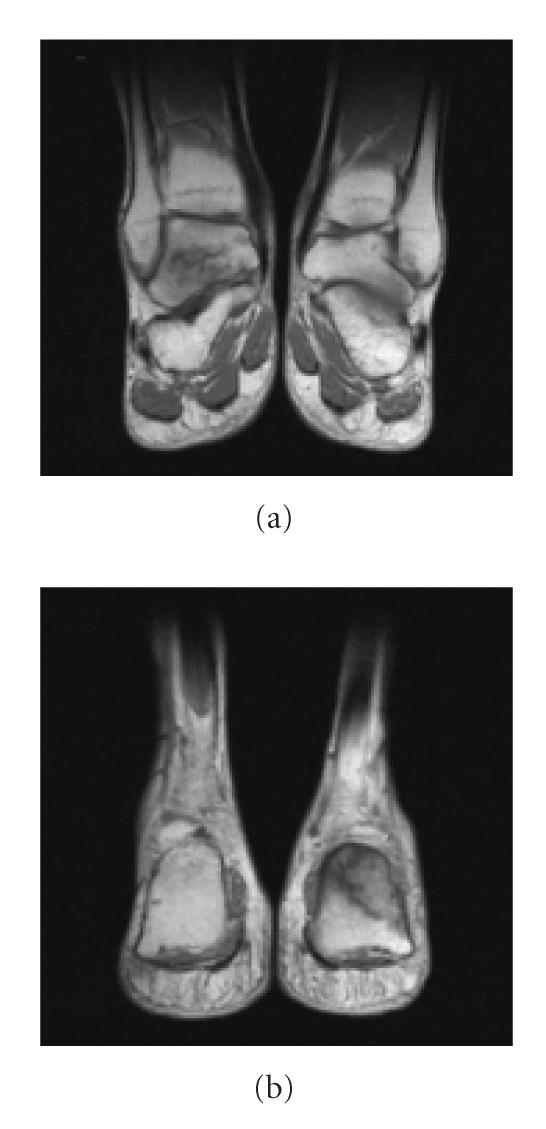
Case 1: T1-weighted magnetic resonance (MR) demonstrating stress fractures of (a) right talus and (b) left calcaneum.
